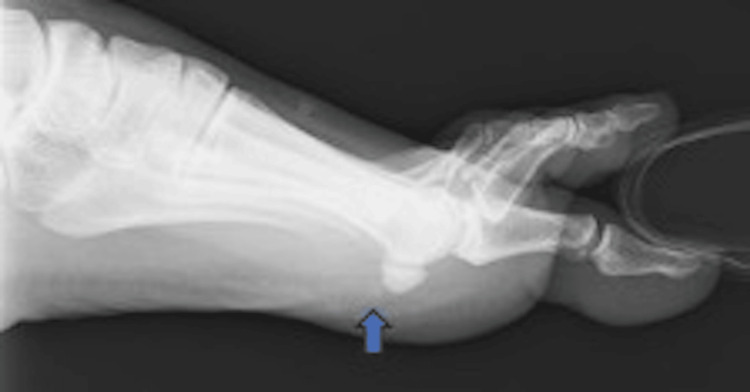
An initial X-ray The arrow shows a cloudy calcification adjacent to the medial sesamoid.
